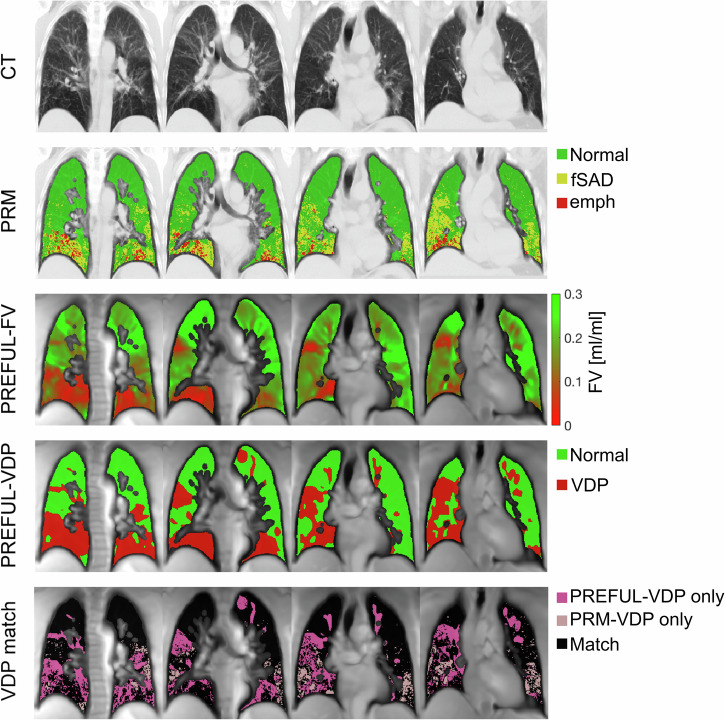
Representative coronal maps of a 60-year-old man with GOLD I disease (FEV_1_ 91% of predicted value, PRM^normal^ 78%, PRM^fSAD^ 19%, PRM^emph^ 4%, PRM^fSAD+emph^ 23%, median PREFUL-FV 0.20, PREFUL-VDP 38%, spatial overlap 69%). First row: CT; second row: parametric response maps (PRM); third row: FV derived by PREFUL MRI; fourth row: ventilation defect map derived by PREFUL MRI; fifth row: black: matching results of both methods, pink: ventilation defect detected by PRM only, purple: ventilation defect detected by PREFUL MRI only
